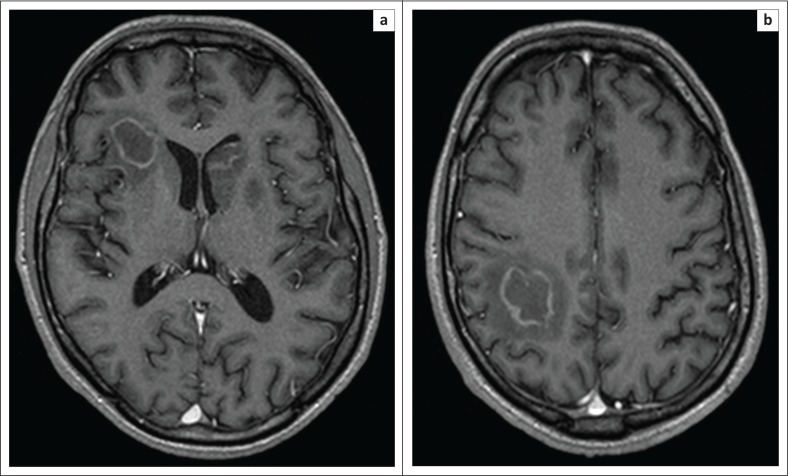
Post-gadolinium T1 contrast enhanced axial slices from MRI brain performed 2 days following the initial clinical presentation. Multiple ring-enhancing lesions are seen within the right frontal (a), left basal ganglia and corticomedullary junction within the right parietal lobe (b). Effacement of anterior horn of the left lateral ventricle is noted (a).
